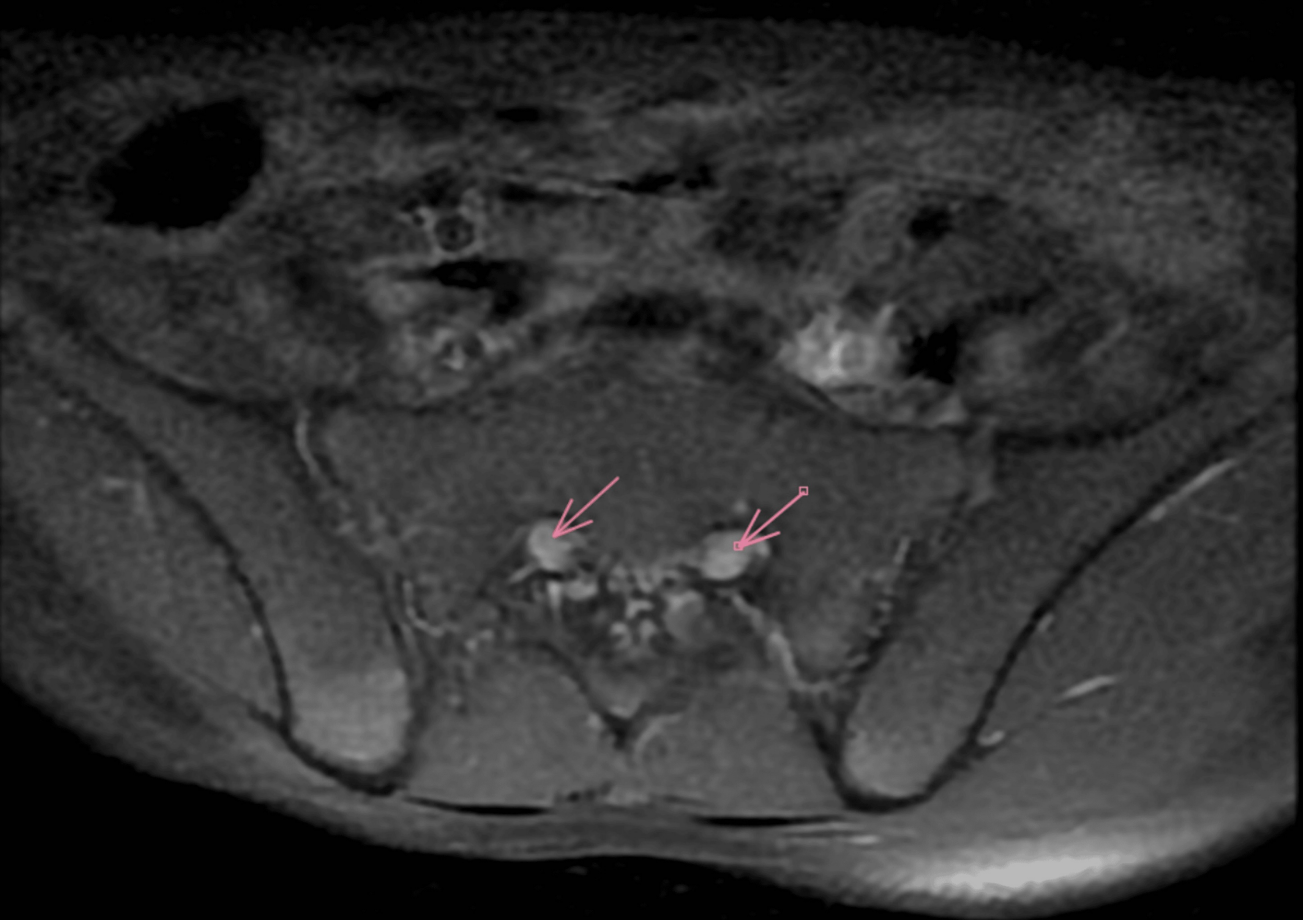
MRI with axial section in T1-weighted images with fat suppression and gadolinium contrast Brain and spinal MRI of our patient demonstrates symmetrical thickening of the lumbar nerve roots with marked enhancement following gadolinium administration. These findings are consistent with Guillain-Barré syndrome.
